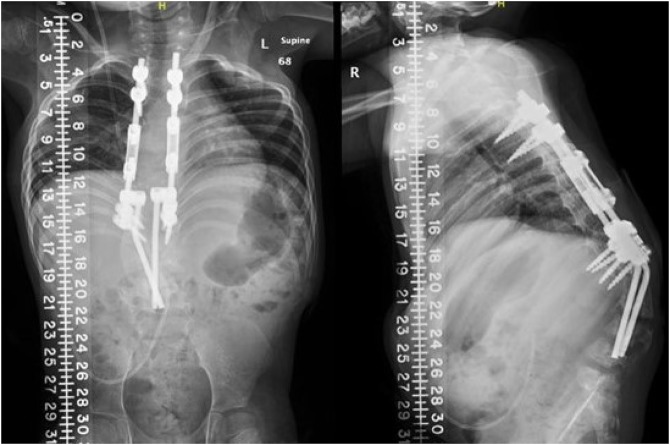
Subsequent AP and lateral radiographs after growing rod expansions at 3 years postoperatively. Proximal migration of the distal construct is noted as the lumbar spine *grows off* the rods.
